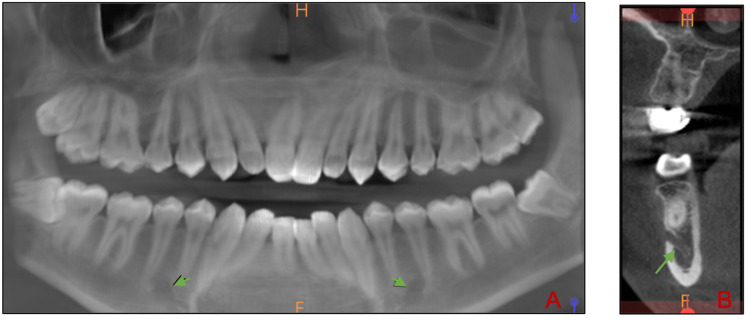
Detection of the anterior loop using CBCT (A) Anterior loop detected bilaterally (green arrows) in the reconstructive panoramic view from CBCT. (B) The cross-sectional slice shows an anterior loop.
